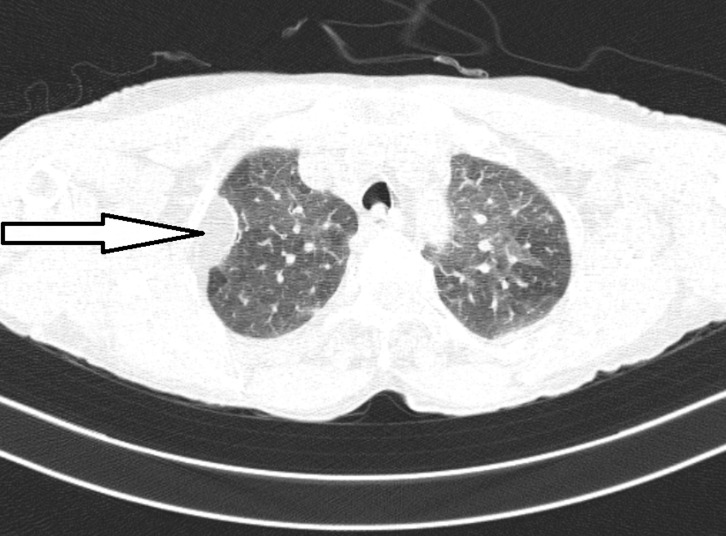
computed tomography showing bilateral ground glass opacities with mosaic attenuation with a white arrow showing homogenous hypodense (-90HU) lesion in pleural space suggestive of pleural lipoma
